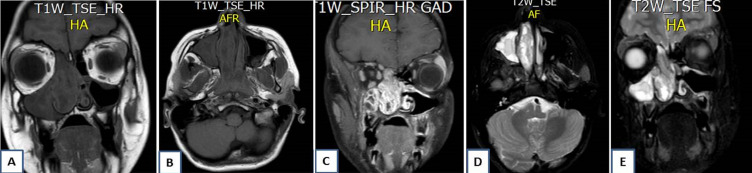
MR images showing an iso-intense T1-weighted sequence right naso-sinusal mass (A, B) with a peripheral enhancing on T1-weighted-gadolinium sequences (C) and “cerebriform” aspect on T2-weighted sequences (D, E)
